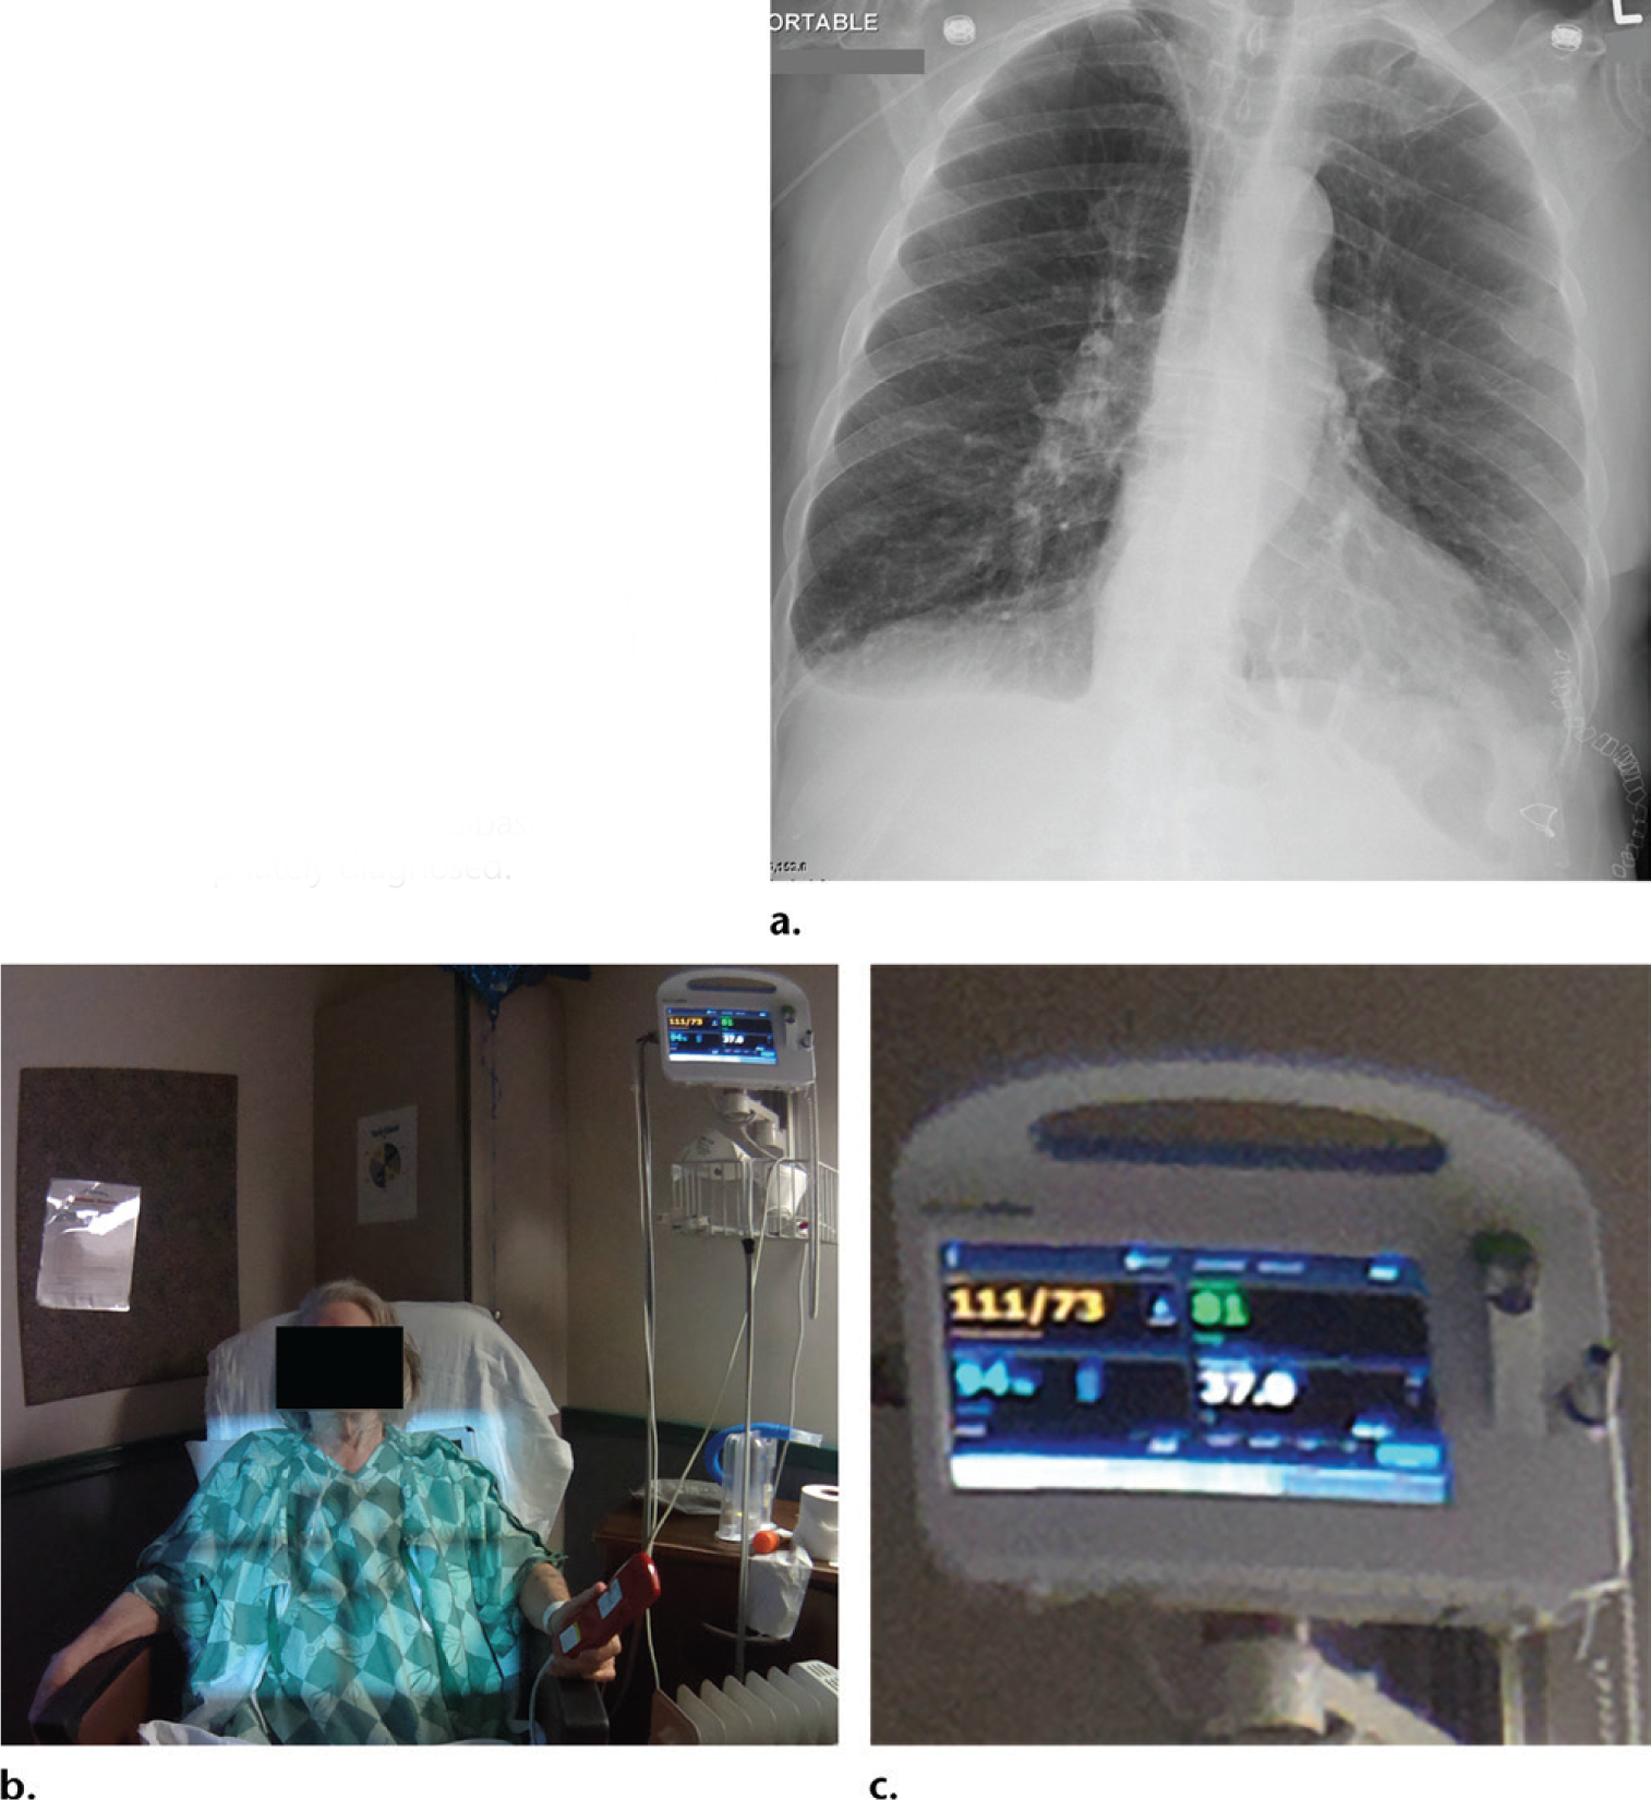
(a) Radiograph obtained to rule out pneumonia shows small pleural effusions and minimal bibasilar airspace opacities. (b, c) Corresponding photograph (b) with a magnified view of the patient’s monitor (c) show the patient’s vital signs (heart rate, 81 beats per minute; blood pressure, 111/73 mm Hg; oxygen saturation, 94%; and temperature, 37.0° C). The patient’s normal temperature indicated that a diagnosis of pneumonia was not correct, and bibasilar atelectasis was appropriately diagnosed.
